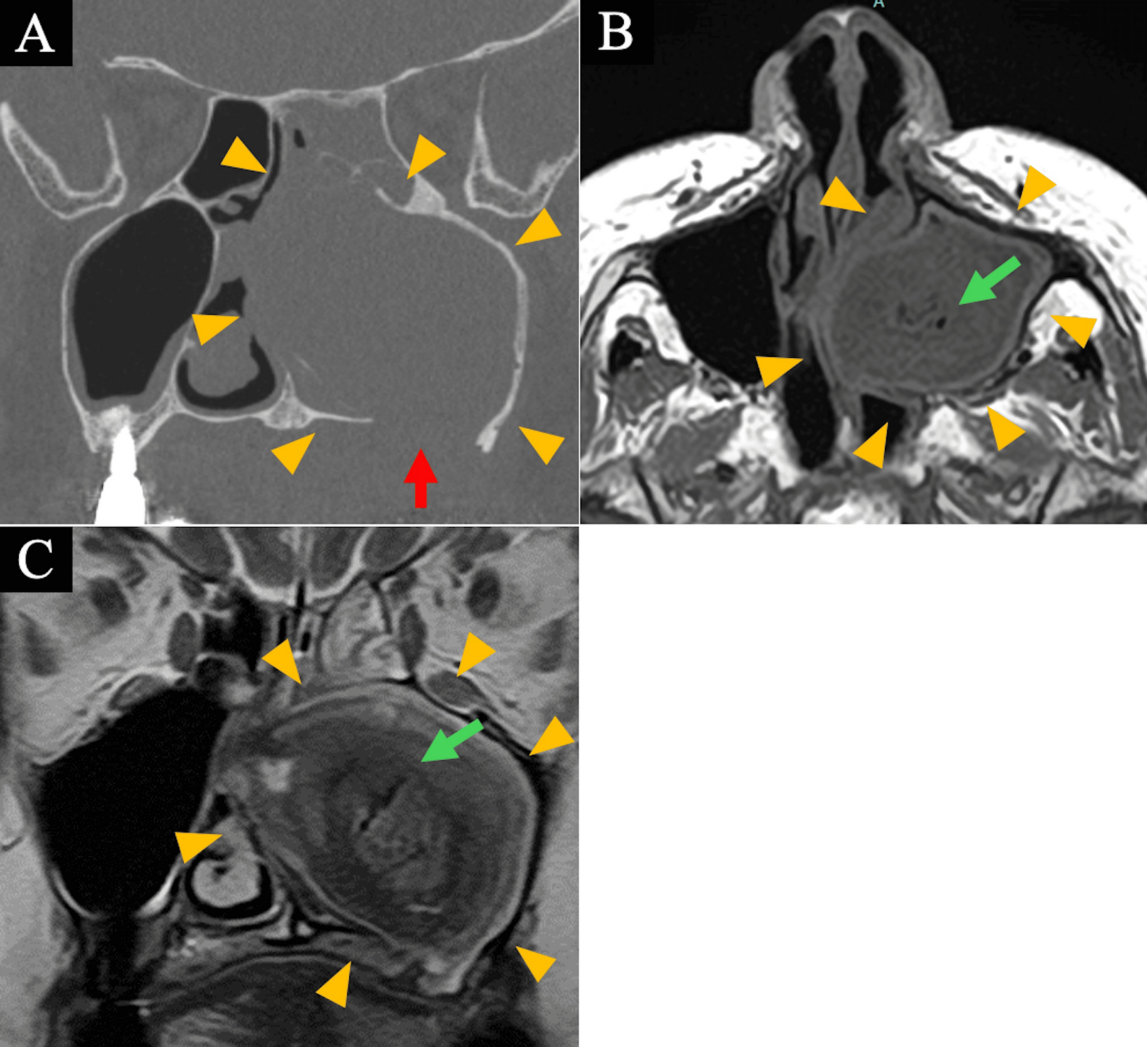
Paranasal imaging findings (A) Coronal CT scan showing an expansile soft-tissue lesion filling the left maxillary sinus with bone resorption at the sinus floor (red arrow). Yellow triangles indicate diffuse soft-tissue opacification within the left maxillary sinus. (B) Axial T1-weighted MRI demonstrating low signal intensity within the lesion. Green arrow indicates internal air retention within the soft-tissue lesion. (C) Coronal T2-weighted MRI showing iso- to low-signal intensity of the lesion, with internal air retention (green arrow).
